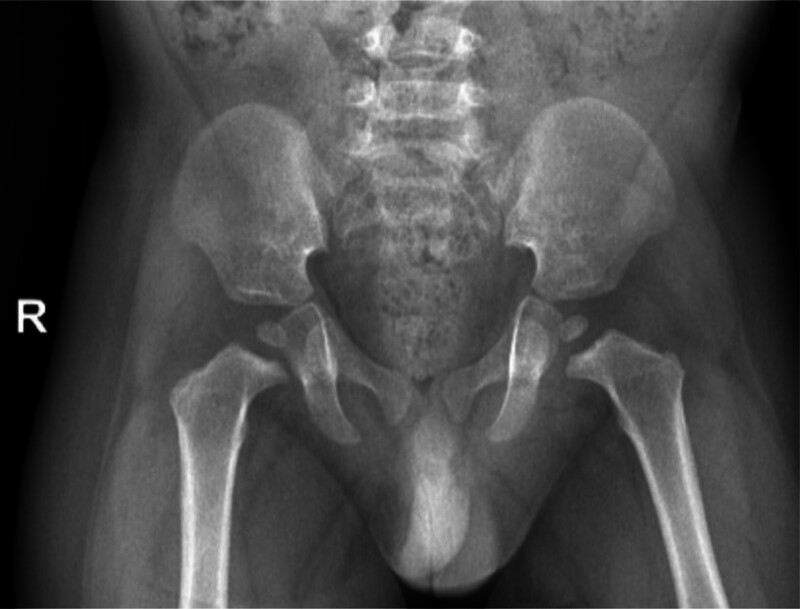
Hip X-ray: bilateral mild coxa vara, widened joint space, irregular femoral head contour with heterogeneous density. Changes in both hip joints warrant evaluation for exclusion of chondrodysplasia.
